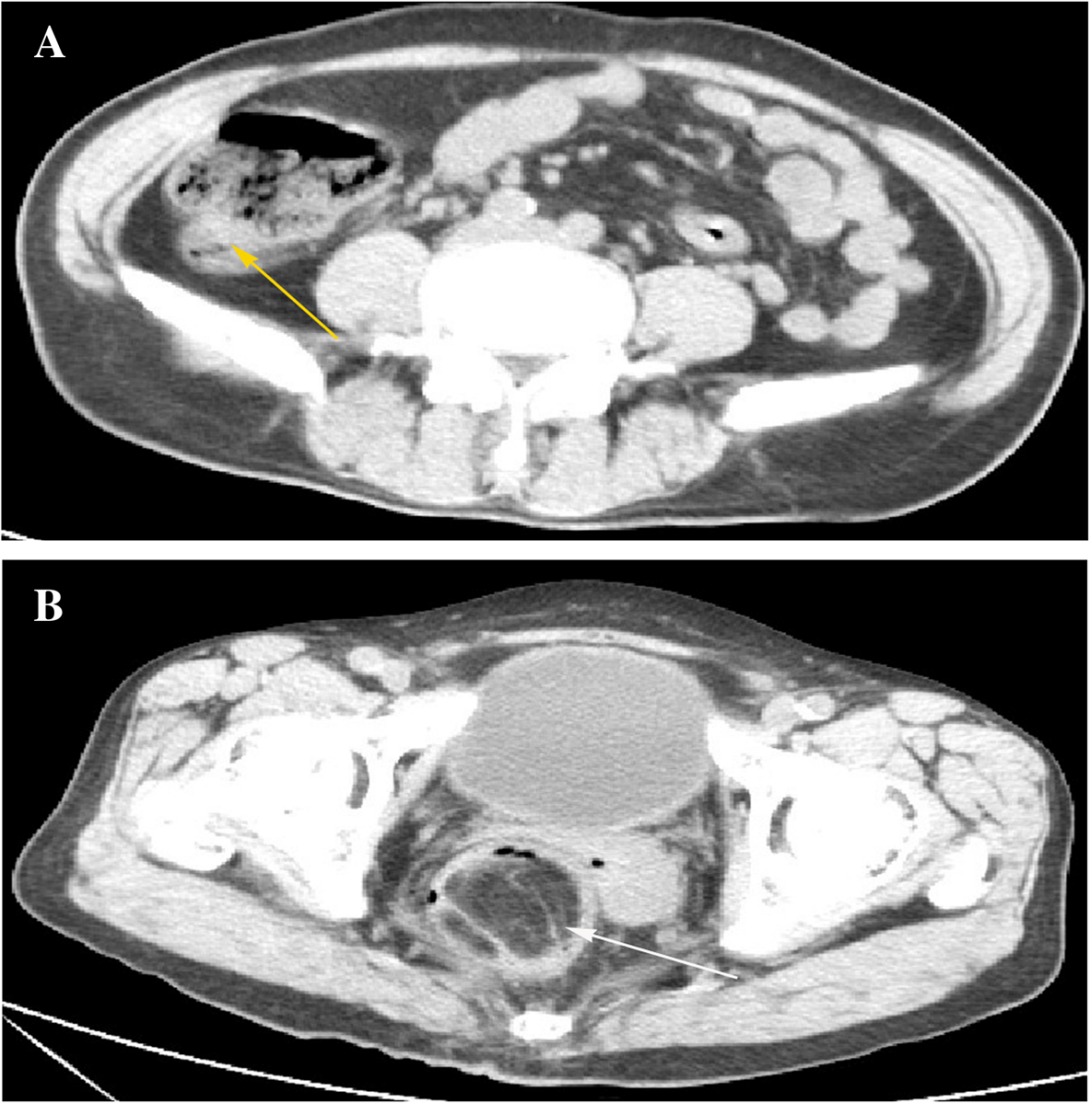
Computed tomography (CT) of the abdomen. (A) CT showed a mass shadow in the ileocecal valve with a maximum size of 2.90 × 3.22 cm (yellow arrow). (B) CT showed the rectum expanded with fat density and space inside a shadow (white arrow).
